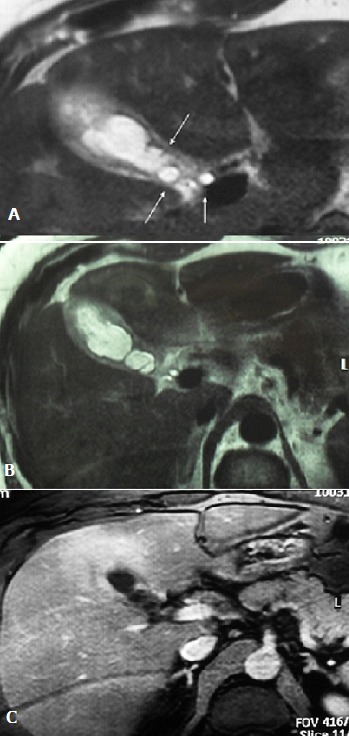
T2-weighted sequence demonstrating daughter cysts in the lumen of gallbladder (A,B); hepatic parenchyma free of cystic lesions (C)
